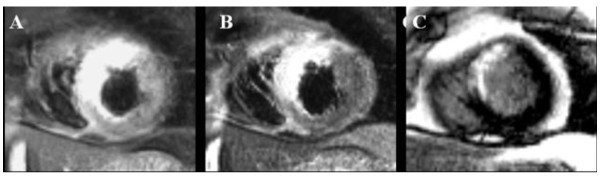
T2-weighted and LGE CMR images. T2-weighted cardiac magnetic resonance (CMR) images acquired by (A) protocol 1 (TE 65msec; slice thickness 15 mm) and (B) protocol 2 (TE 100 msec; slice thickness 8 mm) and (C) LGE CMR in a 62-years-old male with anteroseptal infarction due to occlusion of the left anterior coronary artery. The myocardial infarct size was 9%LV (left ventricle). The myocardial area at risk was 31%LV measured by protocol 1 and 28%LV by protocol 2.
